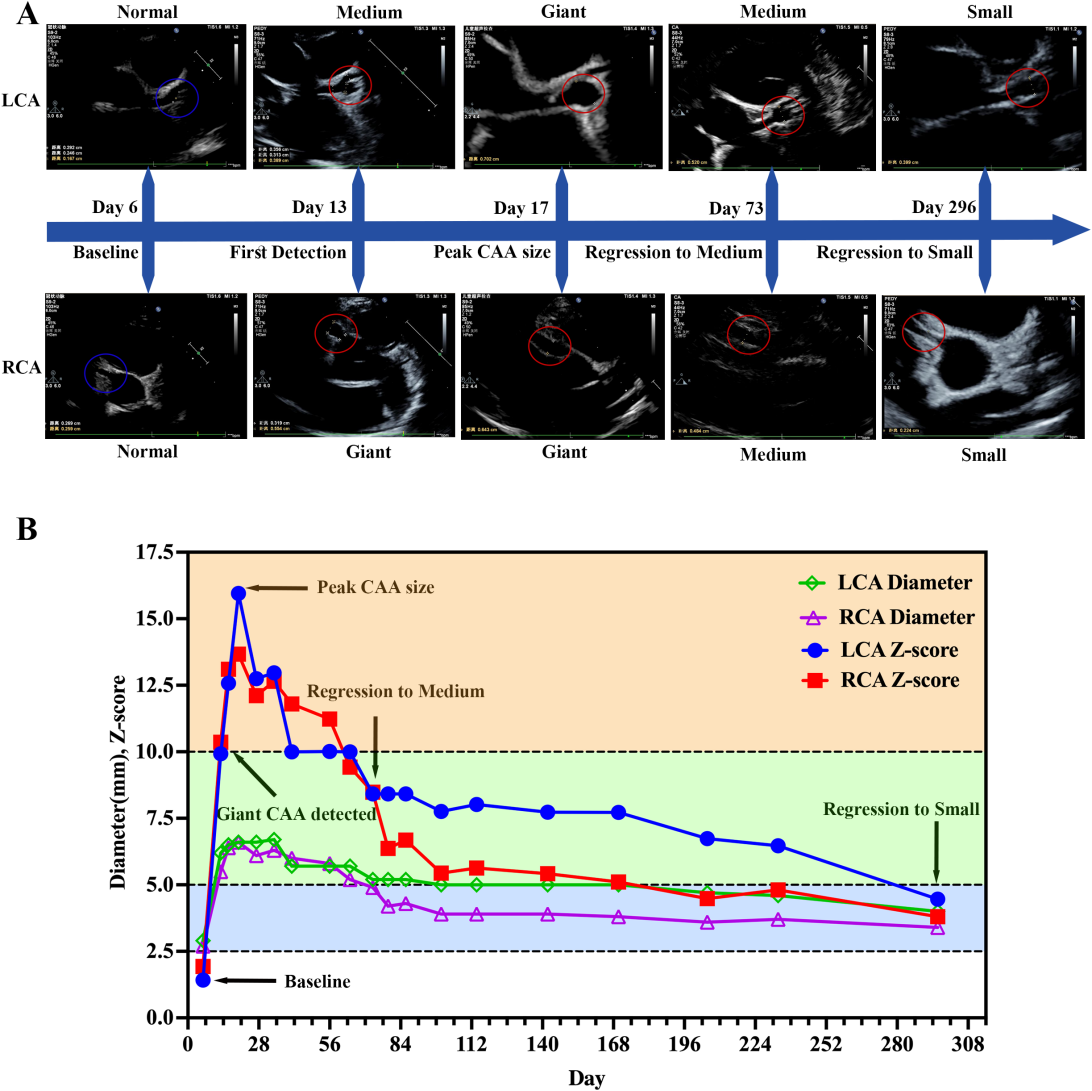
Assessment of coronary artery aneurysms (CAAs) progression and regression. (A) Serial echocardiography images of the left coronary artery (LCA) and right coronary artery (RCA) at critical milestones: baseline (Day 6), initial diagnosis of giant CAA (Day 13, Z score ≥ 10), peak dilation (Day 16), and progressive regression (Days 73 and 296). Baseline normal segments are indicated by blue circles; dilated segments are highlighted by red circles. (B) Dynamic trends in LCA/RCA diameters and Z-scores, highlighting the transition from giant to small CAA stages.
